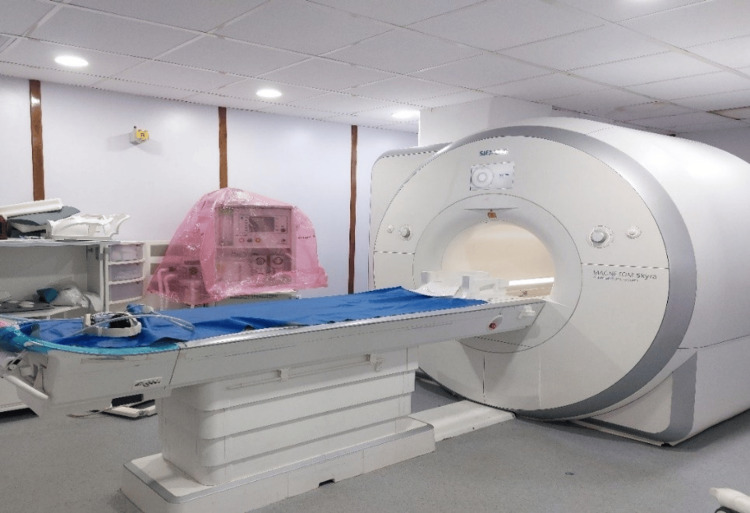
Siemens 3.0-Tesla MRI scanner (Skyra, Erlangen, Germany) used for all MRI examinations in this study. The scanner is equipped with Explorer gradients (maximum gradient strength = 45 mT/m; slew rate = 200 mT/m/ms) and a phased-array multi-channel body coil. MRI = magnetic resonance imaging
